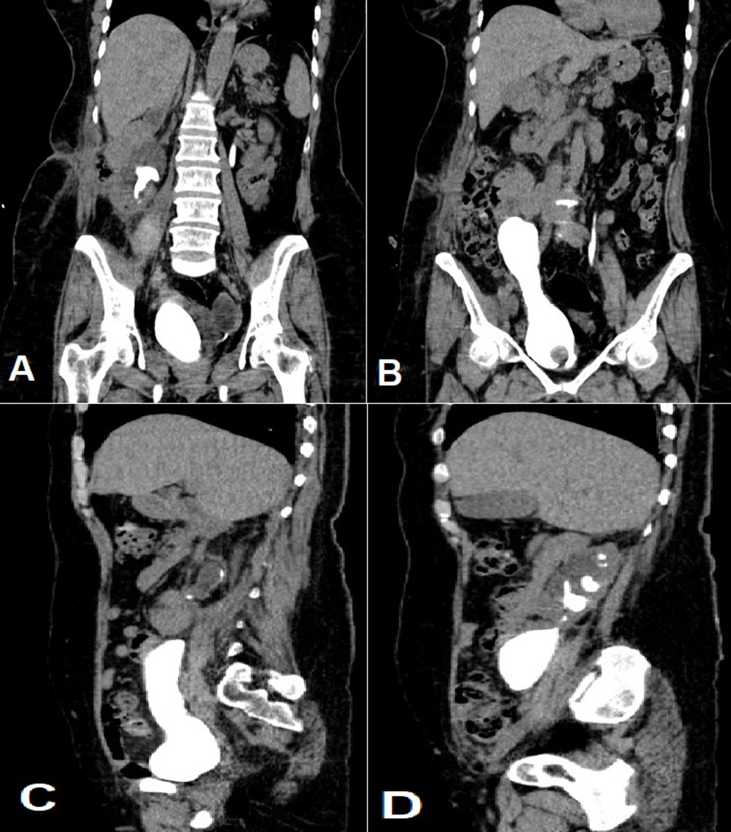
Computed Tomography (CT) cystography after Calicovesicostomy surgery. Anastomosis site was open and no stenosis or obstruction was seen. Coronal view (A–B), Sagittal view (C–D).
